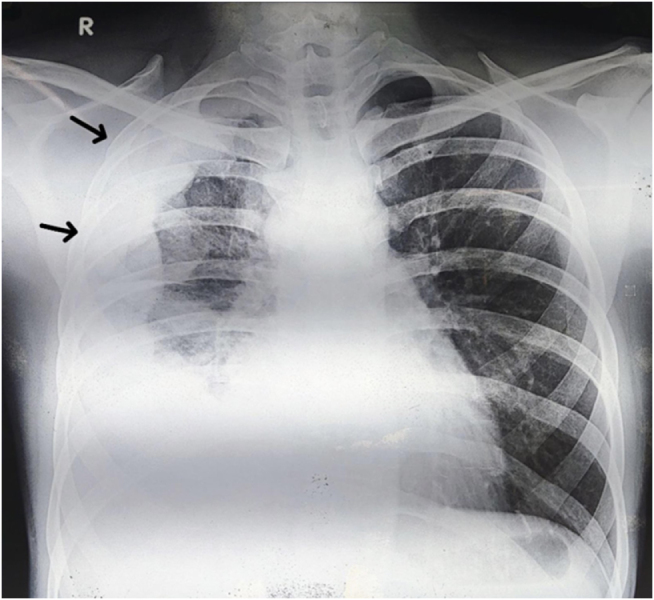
Chest radiograph demonstrating a right-sided pleural effusion with associated ipsilateral volume loss and irregular pleural margins (black arrows), raising suspicion for pleural-based pathology.
